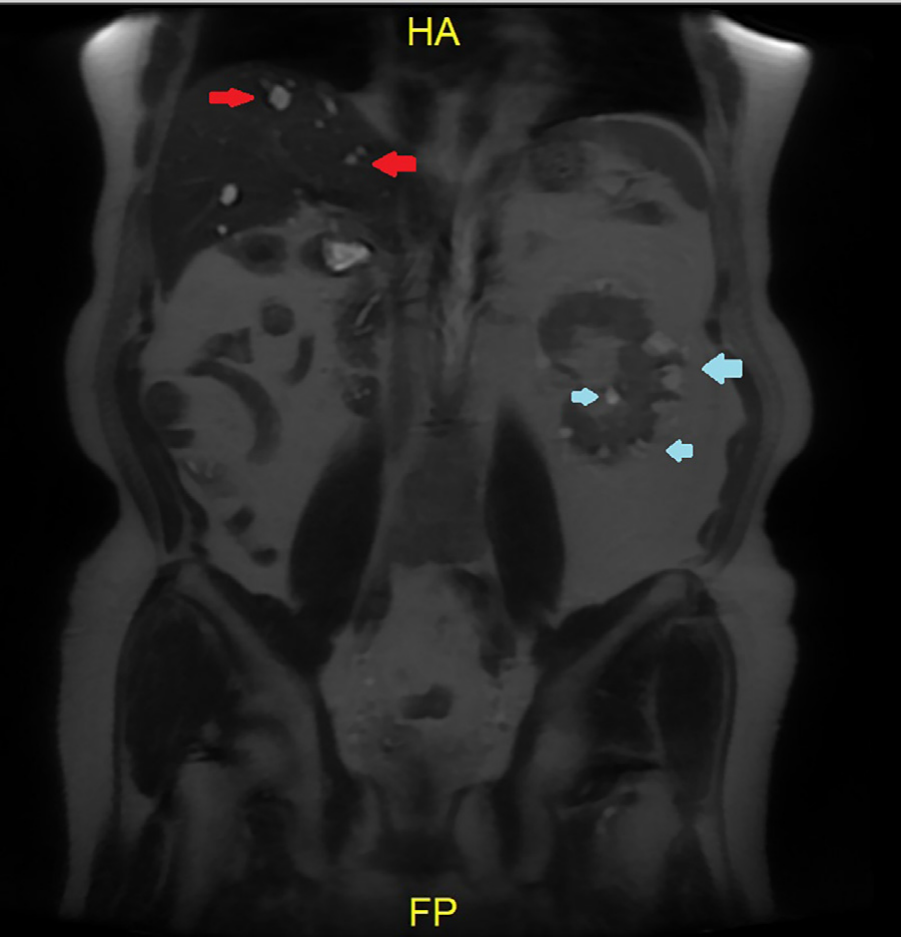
Follow-up MRI revealed multiple cysts on the liver (red arrow) and kidney (blue arrow) with the largest diameter of 2 cm and no sign of a residive mass.
